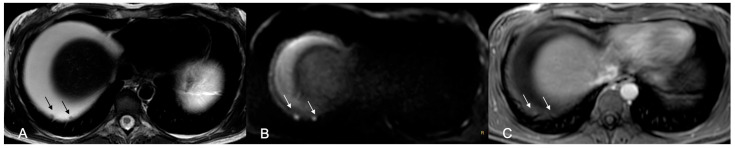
Axial MR images at the level of the liver dome. T2W (A), DWI b 1000 (B), and T1W post-contrast with fat saturation (C) images demonstrate two small up to 5 mm solid nodules on the right hemidiaphragm (arrows in (A)), with restricted diffusion (B) and contrast enhancement (C).
